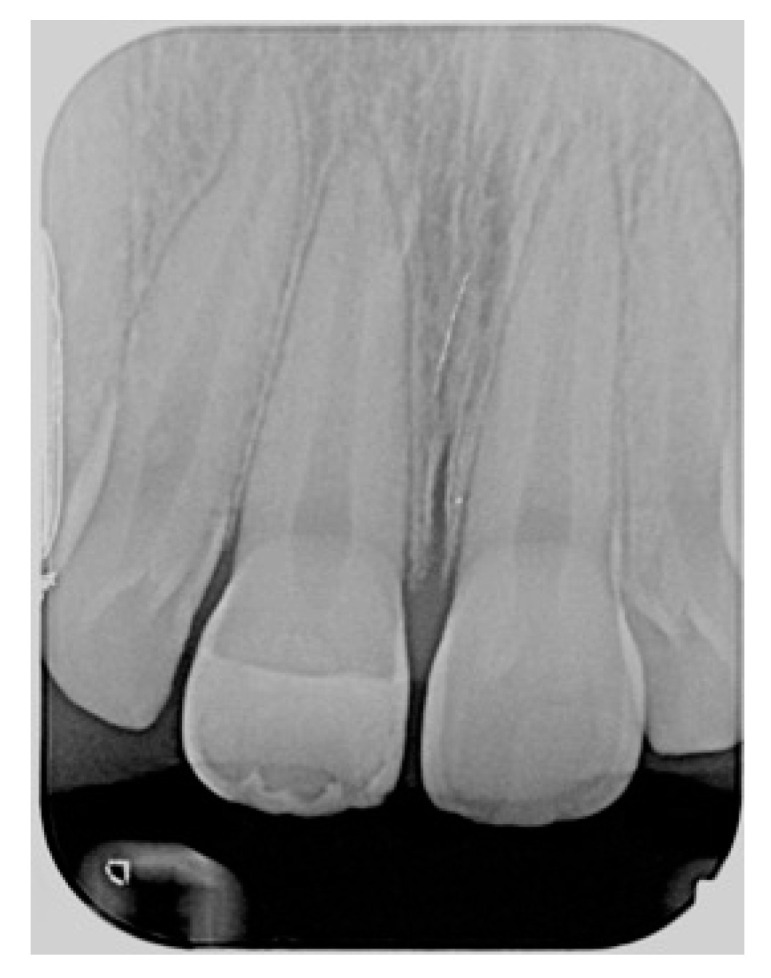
Five years post-operative x-ray.
